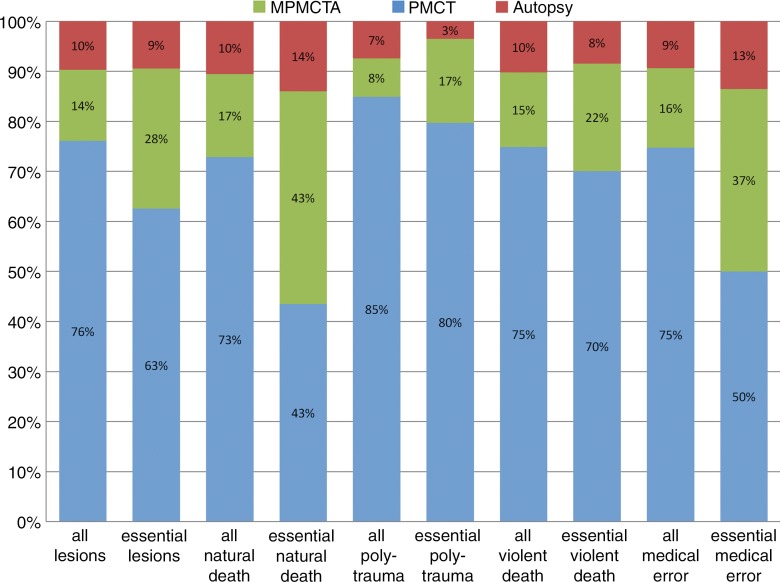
Graph of additional postmortem findings. (a) Additional findings obtained by using imaging with autopsy. Autopsy findings as a percentage of all findings are in red. Additional findings not observed at autopsy but identified at postmortem CT are in blue. Green indicates findings undiscovered at both autopsy and postmortem CT but detected at postmortem CT angiography. There is a relatively high effect of postmortem CT in polytrauma evaluation and of postmortem CT angiography in medical errors and natural death. (b) Additional findings obtained by using angiography with postmortem CT, and then by performing an autopsy. Postmortem CT findings as a percentage of all findings are in blue. Additional findings detected by using postmortem CT angiography are in green. Finally, findings only detected at autopsy are in red. Notice the relatively high effect of autopsy in medical errors and natural death, and the low effect in evaluation of polytrauma.
